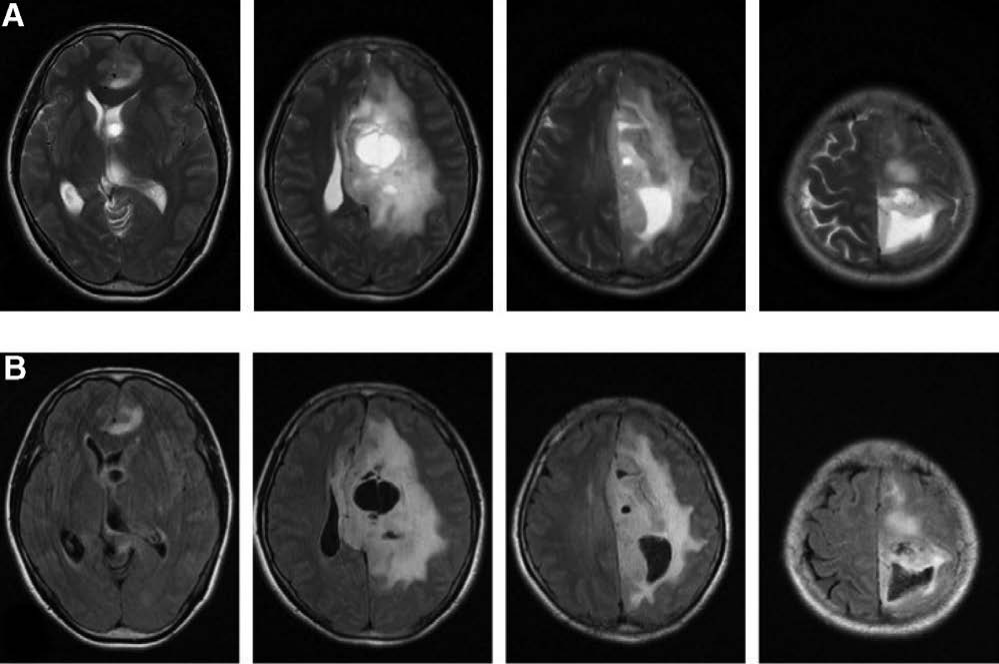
The MRI of pre-operation. (1) T2 (A) and T2 flair (B). (2) Left intracranial space-occupying lesion in frontal lobe, parietal lobe, and thalamus. (3) Midline displacement of cerebral hemispheres and subfalcine hernia. MRI = magnetic resonance imaging.
